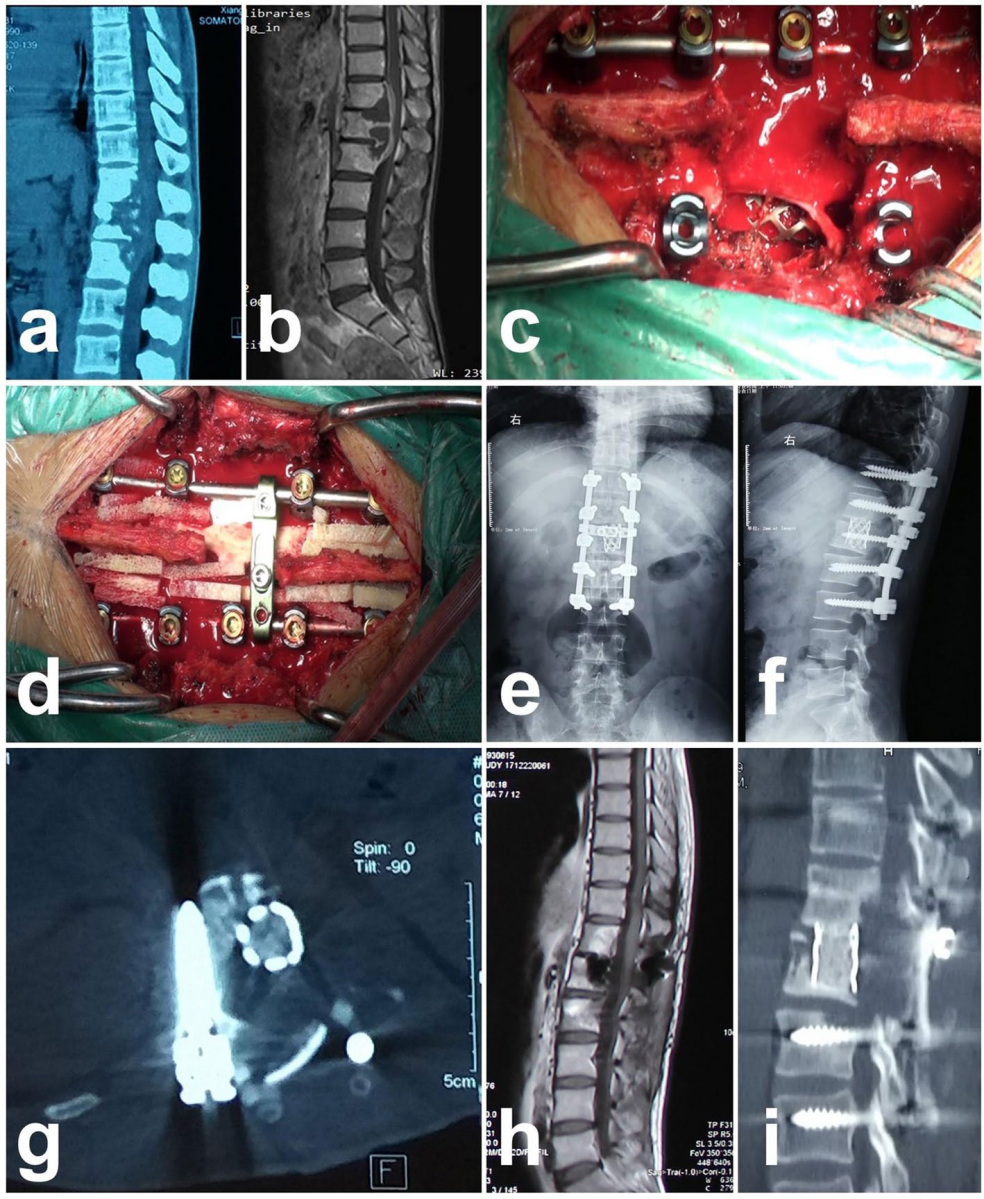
A 26-year-old male, infected with tuberculosis in the T12-L2 region. a: preoperative CT showed T12, L1, L2 region of the vertebral body, and intervertebral disc destruction. b: preoperative MRI image shows tuberculosis lesions in the spinal canal and a dural sac compression; c: the image shows the position of implantation of titanium mesh. d: the picture shows the laminar reconstruction using allogeneic bone. e and f: the postoperative x-ray images show good internal fixation, the implanted titanium mesh located at interbody bone defect area; g: postoperative CT image indicates that the titanium mesh was situated between the vertebral bodies, and the position was proper; h: postoperative MRI image shows a complete relief of spinal cord compression; i: CT sagittal view shows bone graft fusion around titanium mesh during postoperative follow-up
